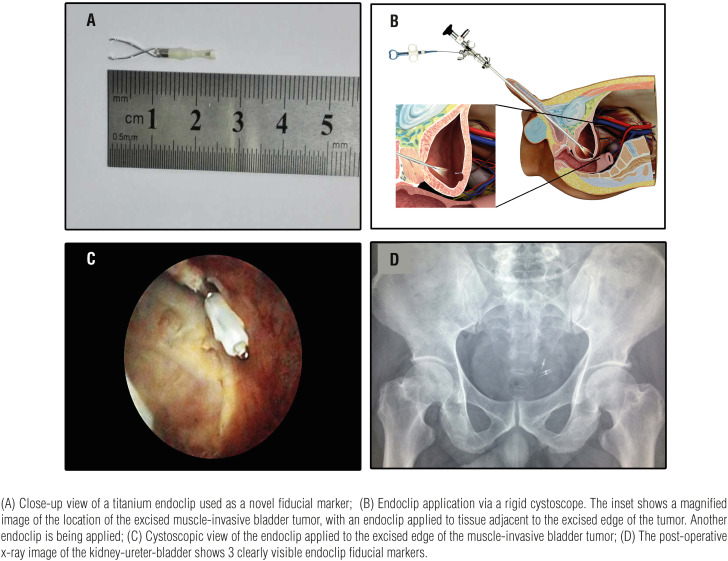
Endoclip Application and follow-up. (A) Close-up view of a titanium endoclip used as a novel fiducial marker; (B) Endoclip application via a rigid cystoscope. The inset shows a magnified image of the location of the excised muscle-invasive bladder tumor, with an endoclip applied to tissue adjacent to the excised edge of the tumor. Another endoclip is being applied; (C) Cystoscopic view of the endoclip applied to the excised edge of the muscle-invasive bladder tumor; (D) The post-operative x-ray image of the kidney-ureter-bladder shows 3 clearly visible endoclip fiducial markers.
